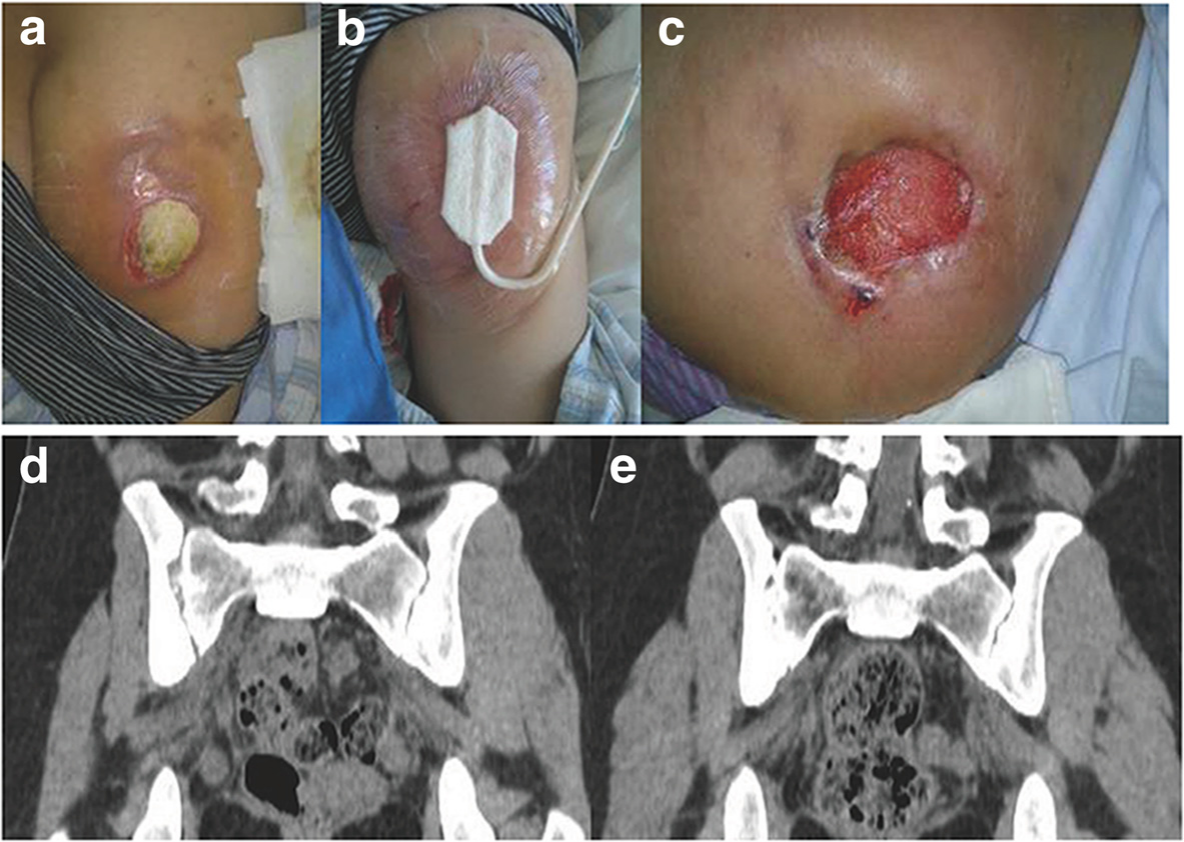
a Sinus and wound in the left buttock. b Management with NPWT. c Formation of fresh granulation tissue after NPWT. d CT scan of sacroiliac joints: erosive changes involving the right sacroiliac joint. e CT scan demonstrating bony fusion 30 months after NPWT and anti-tubercular therapy
